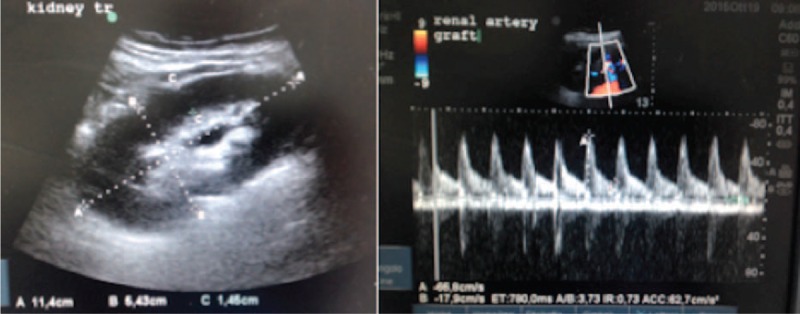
Graft sonography performed on the fourth postoperative day demonstrates near-complete resolution of hydronephrosis with a normal pulsatility index.
